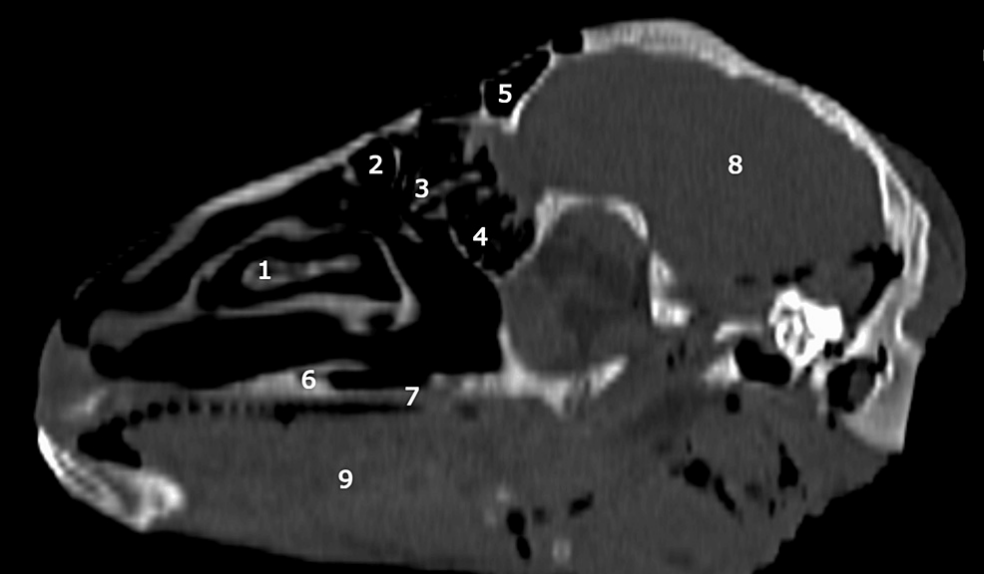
Sagittal CT scan: lateral nasal wall with a high degree of pneumatization of the nasal turbinates and ethmoid sinus 1: inferior turbinate; 2: superior turbinate;3: head of middle turbinate; 4: ethmoidal labyrinth;5: frontal sinus 6: the palatine process of incisive bone; 7: hard palate; 8: brain; 9: body of the tongue.
